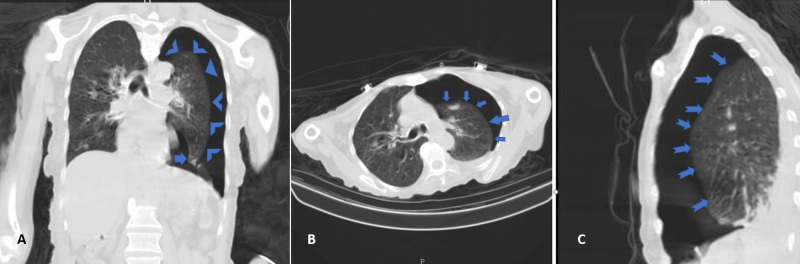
CT chest after removal of the incorrectly placed NGT (A) Coronal plane, (B) axial plane, and (C) sagittal plane CT chest after removal of the incorrectly placed NGT, showing the extent of left-sided PTX. NGT, nasogastric tube; PTX, pneumothorax.
